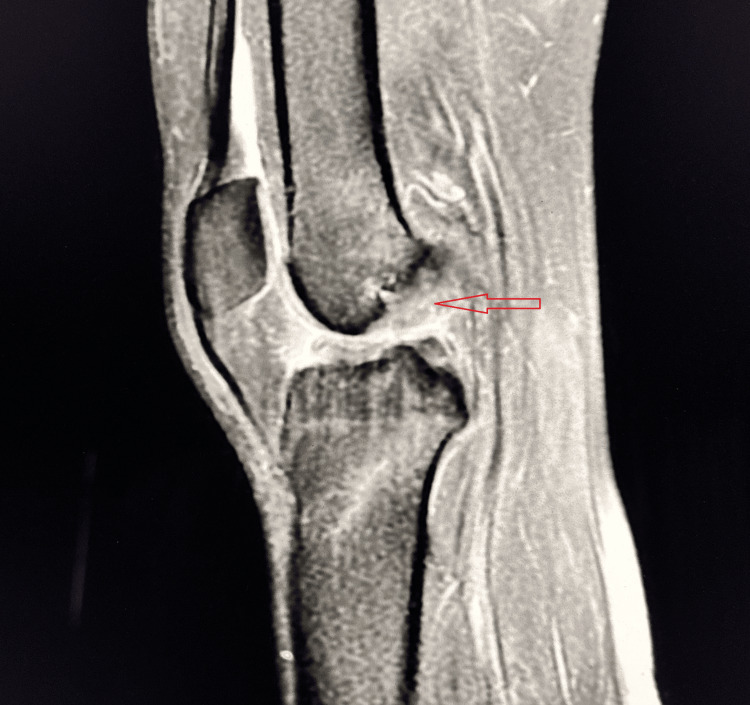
Grade III (complete) tear of the ACL Sagittal PD-FS MRI shows complete discontinuity of ACL fibers with marked hyperintensity, consistent with a complete Grade III ACL rupture (red arrow). Moderate knee joint effusion is also present. ACL, anterior cruciate ligament; PD-FS, proton density fat-suppressed
